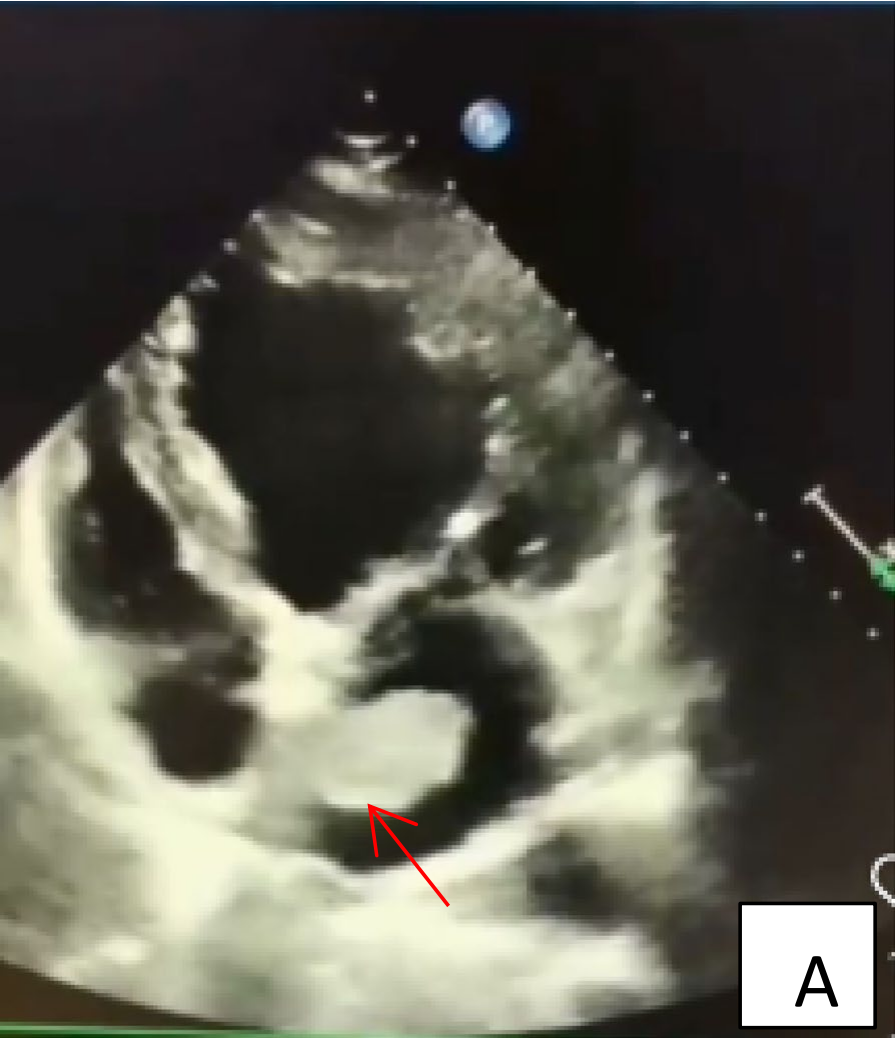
Preoperative echocardiographic findings. Transthoracic four-chamber view echocardiograph of the hemangioma (arrow), showing an irregularly shaped mass attached with a 5 mm- wide stalk to the antero-septal wall of the left atrium
